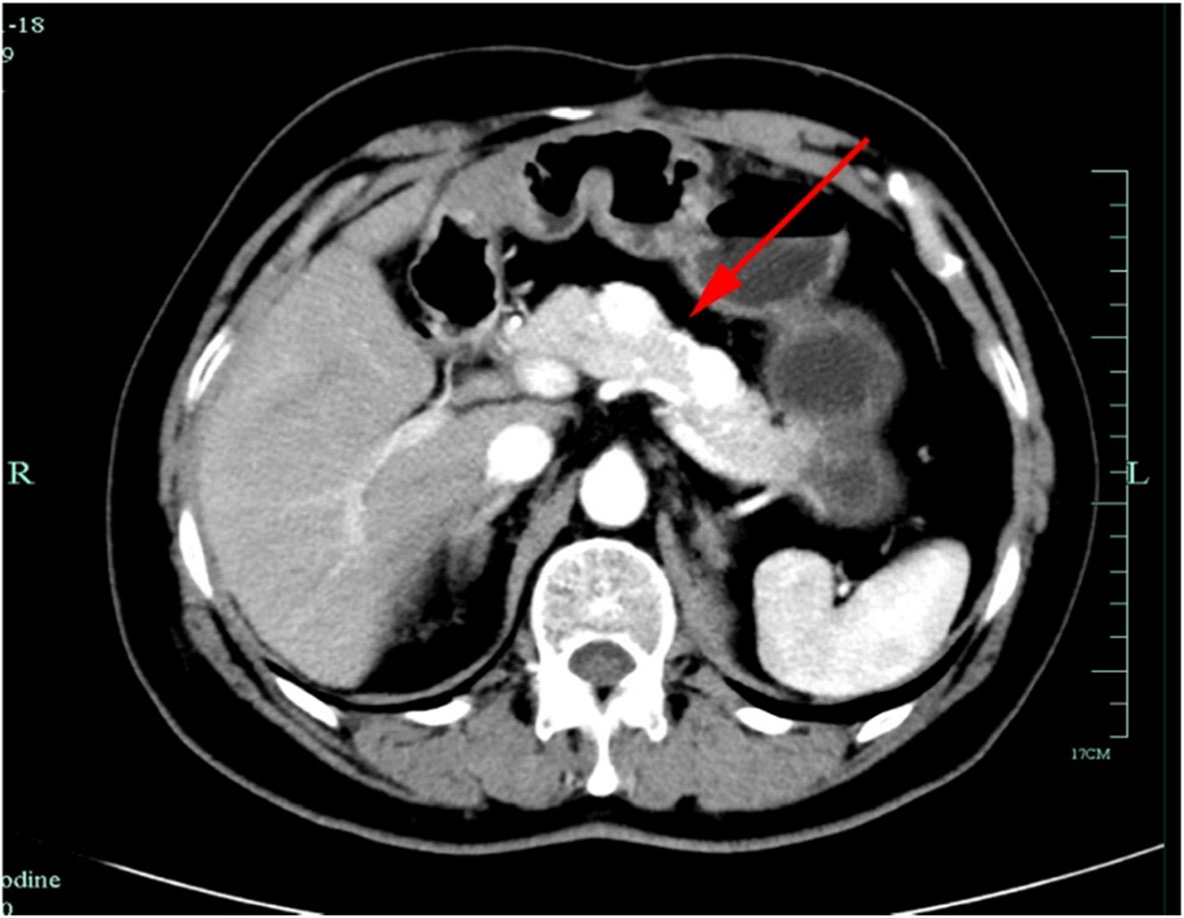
Enhanced computed tomographic scan showing a hypervascular lesion in the pancreas (*red arrow*)
